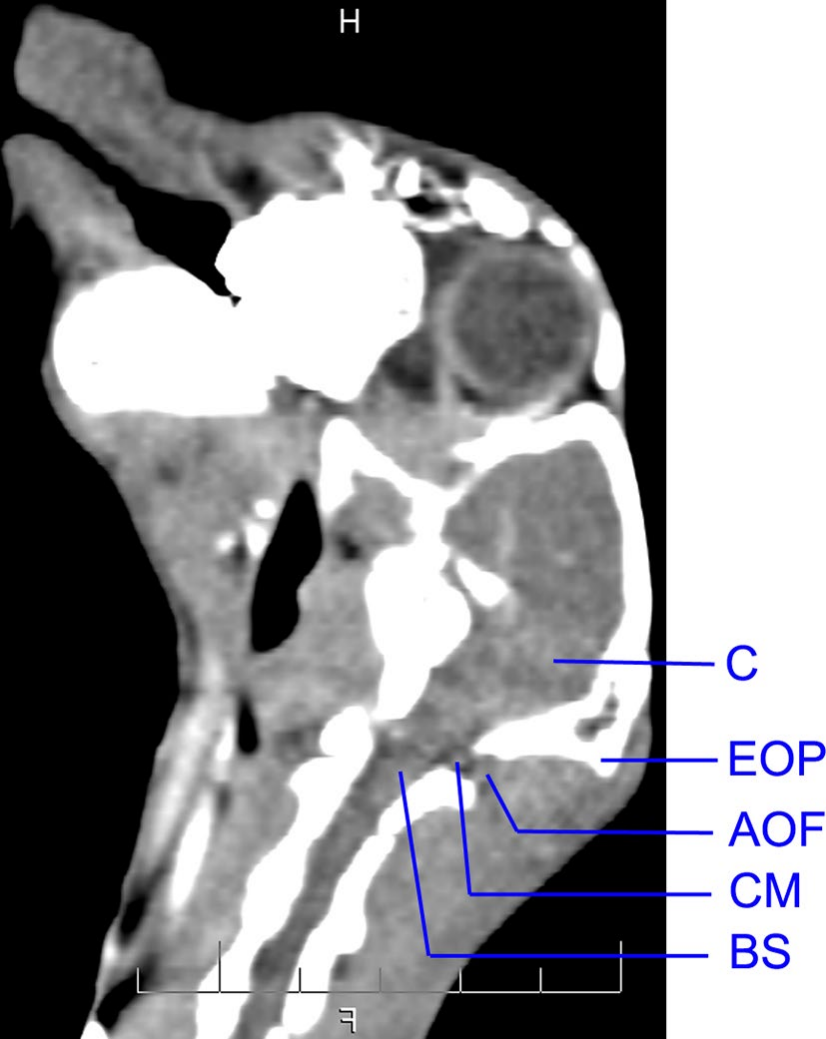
Cisterna magna and atlano-occipital fascia in CT sagittal image. *C* cerebellum, *EOP* external occipital protuberance, *AOF* atlano-occipital fascia, *CM* cisterna magna, *BS* brain stem
